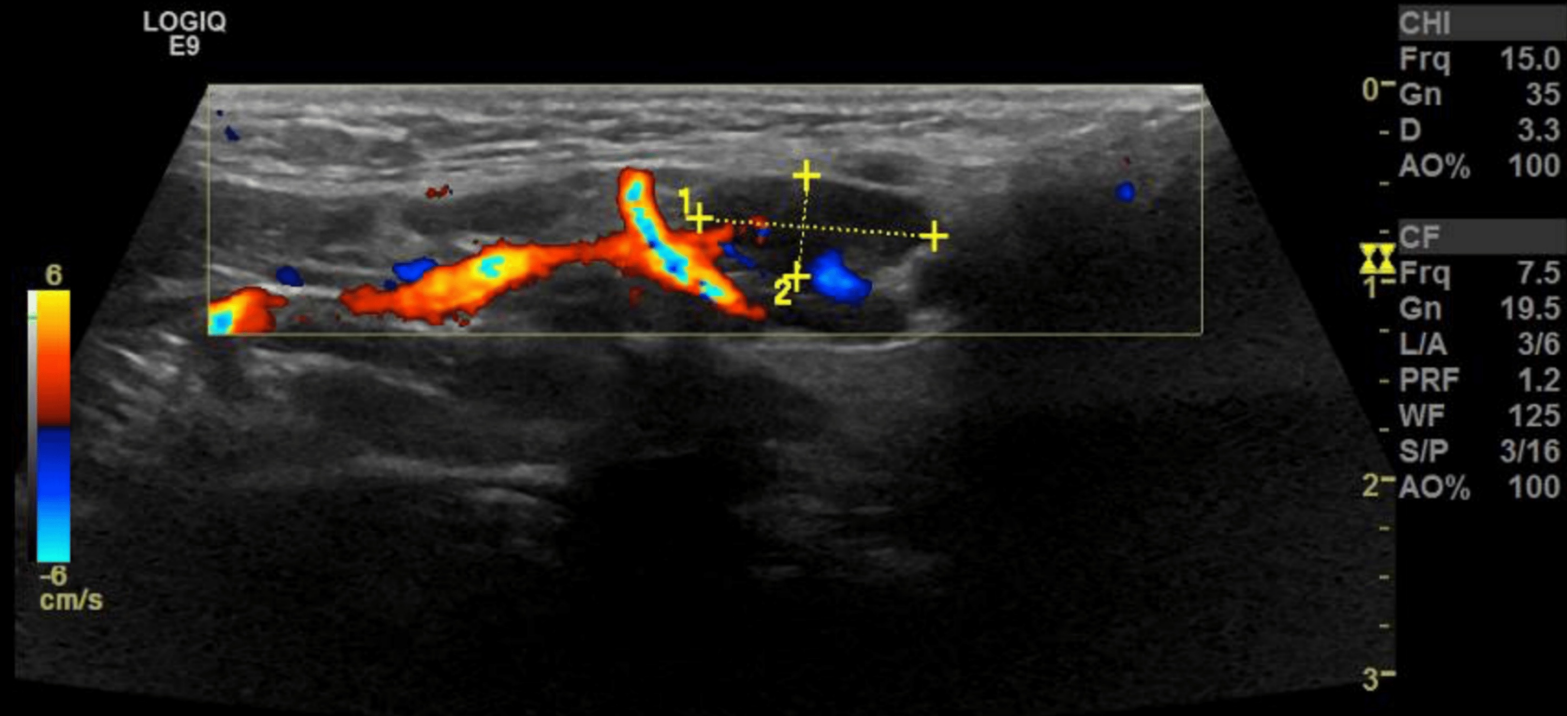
Ultrasound of the neck demonstrating a left submandibular lymph node measuring 1.2 × 0.51 cm Ultrasound image of the left submandibular region showing a well-defined lymph node measuring 1.2 × 0.51 cm in maximal dimensions. No sonographic features of abscess formation or extracapsular extension were identified.
